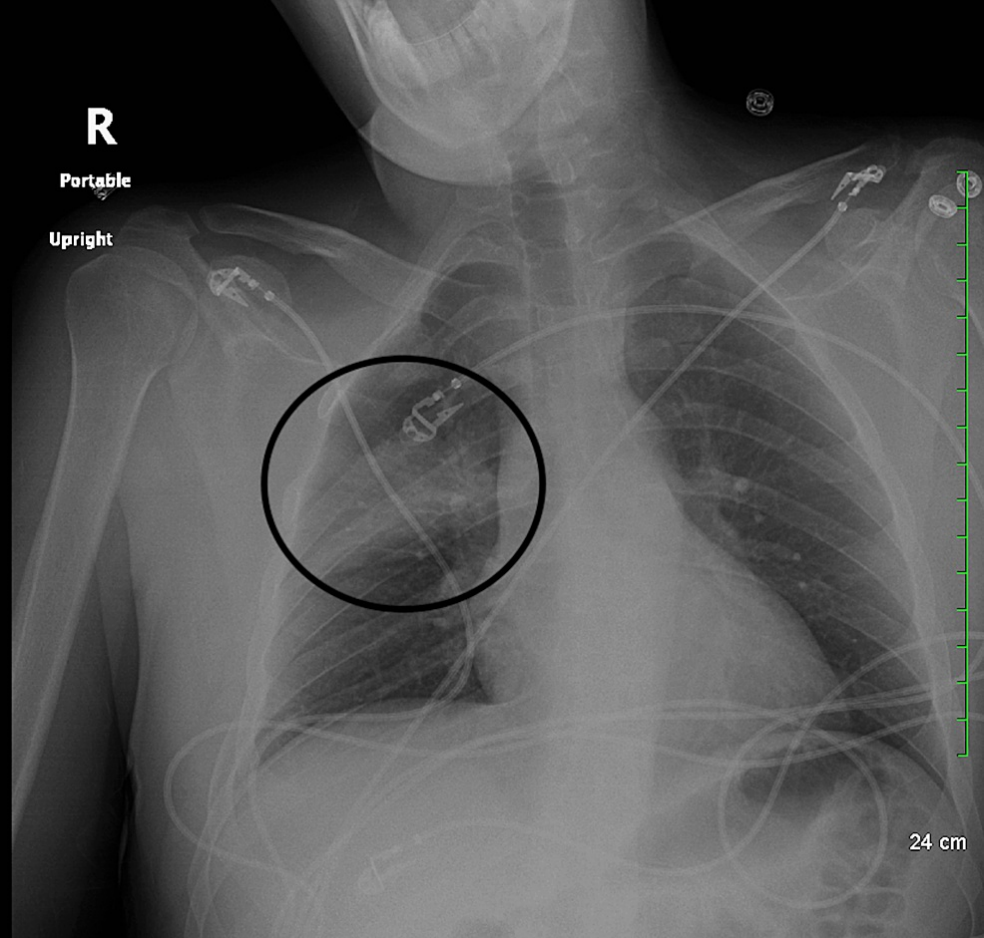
Chest X-ray showing right upper lobe infiltrates
